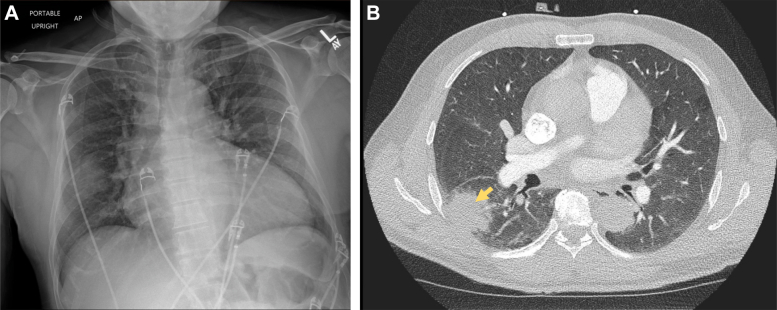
Chest Imaging Performed on Admission (A) Chest radiography showed enlarged cardiac silhouette, low lung volumes, and right mid-hemithorax opacity. (B) Chest computed tomography showed a right lower lobe subpleural consolidation with surrounding ground-glass opacities (yellow arrow), prominent mediastinal and bilateral hilar lymph nodes, and cardiomegaly with left ventricular enlargement.
